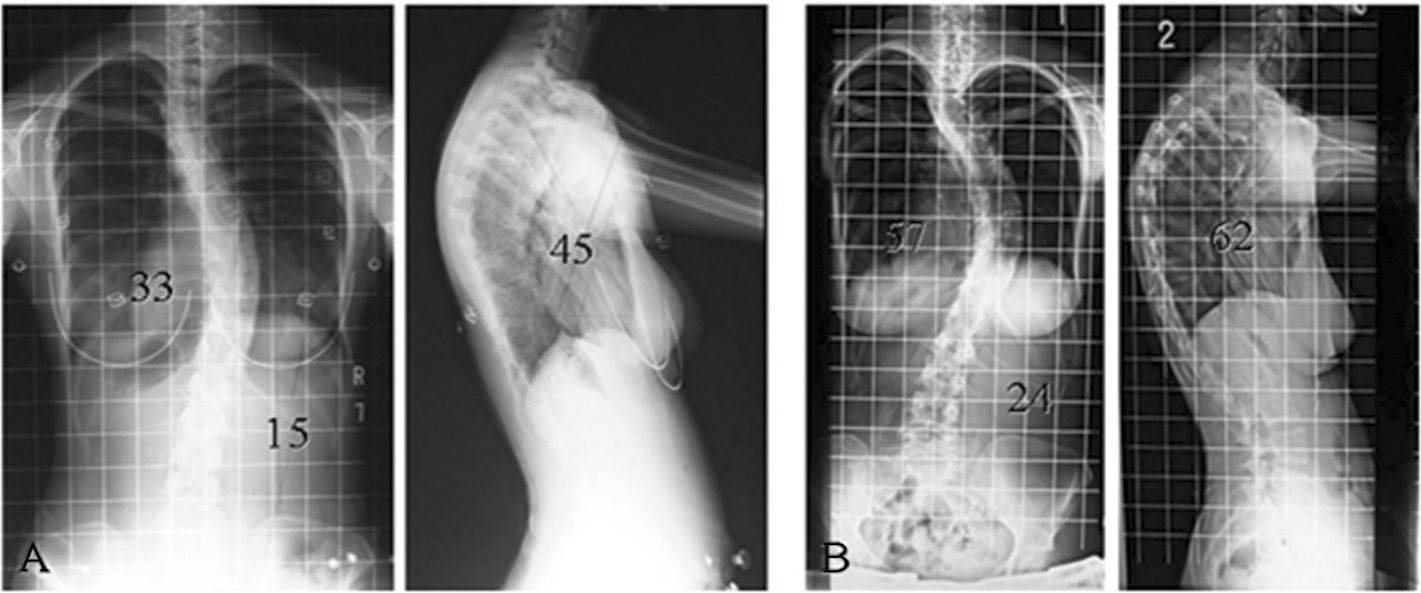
Standing radiographs. (A) Radiographs 1 month after implant removal. (B) Radiographs 18 months after implant removal.
